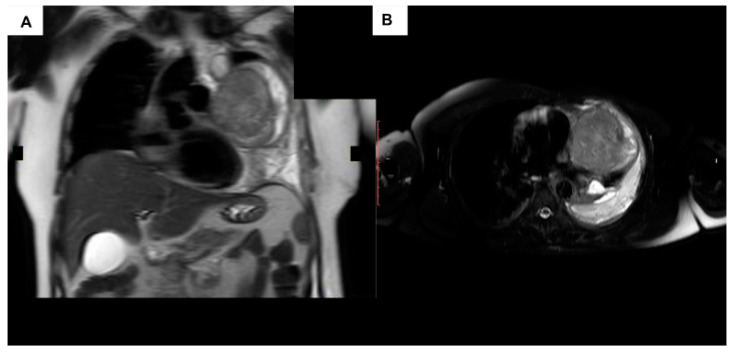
(A) MRI coronal plane; (B) MRI axial plane; Magnetic resonance images for the mass do not recognize infiltration of the great vessels; however, if the establishment of cardiopulmonary by-pass is anticipated, there will be limited exposed area for cannulation.
